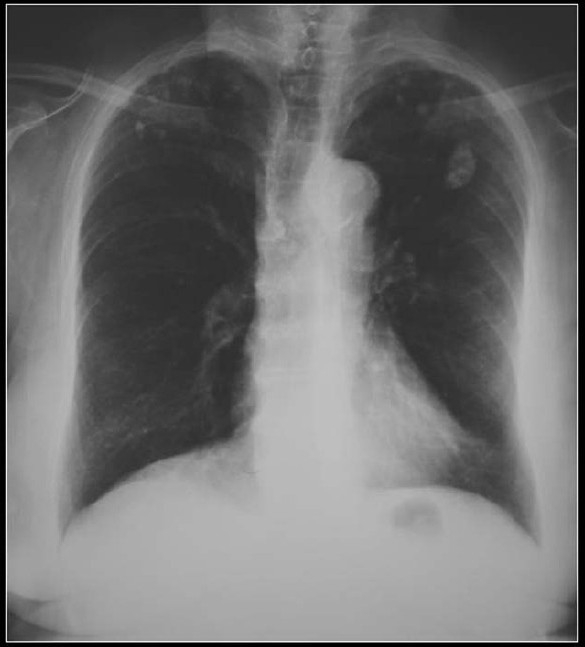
A chest radiograph shows calcification at both the lung apices associated with a right lower paratracheal calcified lymph node due to healed tuberculosis
